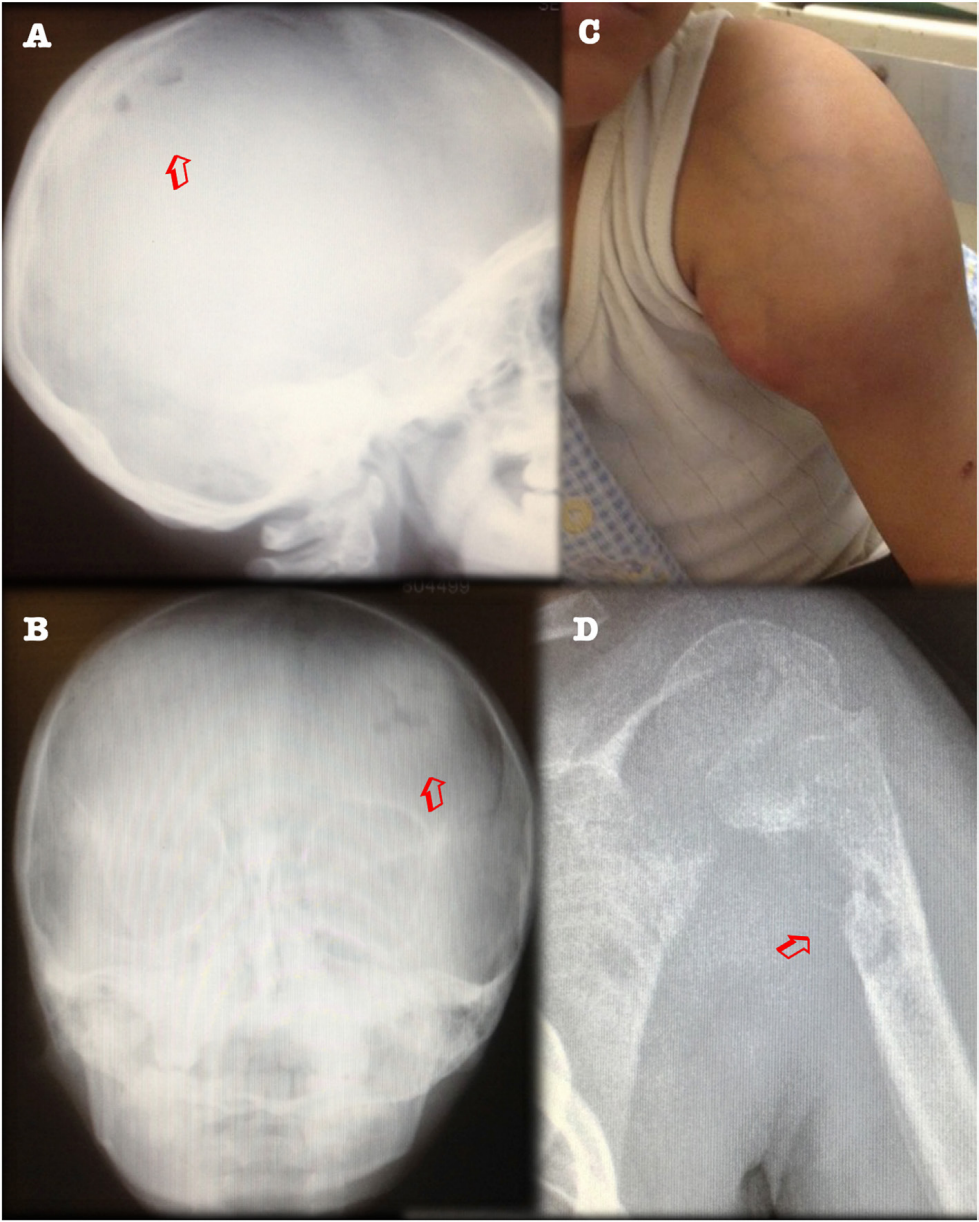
Clinical relapse with upper arm abscess, and new lytic lesions in parietal bone (left panels) and left humerus (right panels). (A) X-ray of skull, lateral view. (B) Posteroanterior view, showing osteolytic lesions (arrows). (C) Swelling of left shoulder over abscess. (D) X-ray of left humerus showing osteolytic lesions (arrow).
